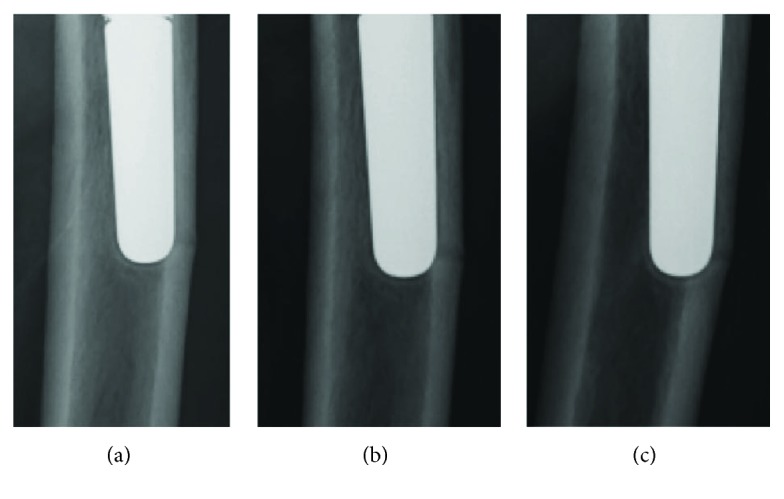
Magnified anteroposterior radiographs of the fracture line, showing gradual clarification of the fracture at six-month follow-up (a), one-year follow-up (b), and two-year follow-up (c).
